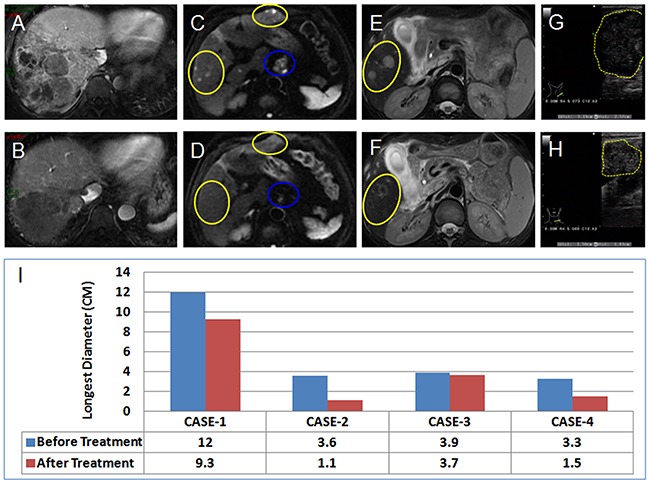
Typical Imaging Changes in Advanced HCC Patients Treated with Apatinib Tyical imaging presentations in 4 patients with advanced HCC (BCLC-C) before (A, C, E, G) and after (B, D, F, H) treatment with apatinib. (A and B) (Case 1), Portal-phase gadolinium-enhanced MR image showing that both a tumor in the liver and an embolus in the vena cava shrank significantly in a PR patient. (C and D) (Case 2), Diffusion-weighted MR imaging showing that intrahepatic metastatic HCC tumors (yellow-circled) disappeared after 4 months of treatment, while metastatic retroperitoneal lymph nodes (blue-circled) shrank dramatically in a PR patient. (E and F) (Case 3), T2-weighted MR image showing significantly decreased tumor activity (yellow-circled) in an SD patient. (G and H) (Case 4), B-ultrasound scan showing a significantly shrunken metastatic infraclavicular lymph node in a PR patient. (I) Changes in tumor size in the longest diameter before and after apatinib treatment (as shown above).
